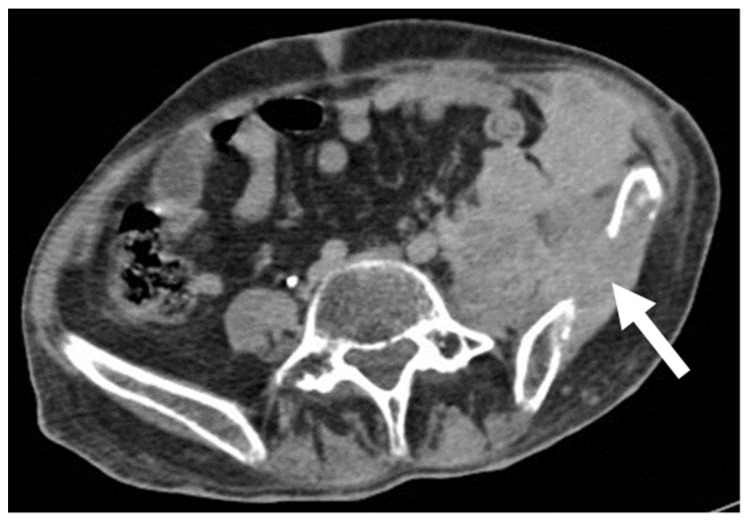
Malignant schwannoma in a 37-year-old man with neurofibromatosis type 1. Portal venous, axial, contrast-enhanced CT scan demonstrates an infiltrative disease, invading the left iliac bone (arrow), and with heterogeneous contrast enhancement.
